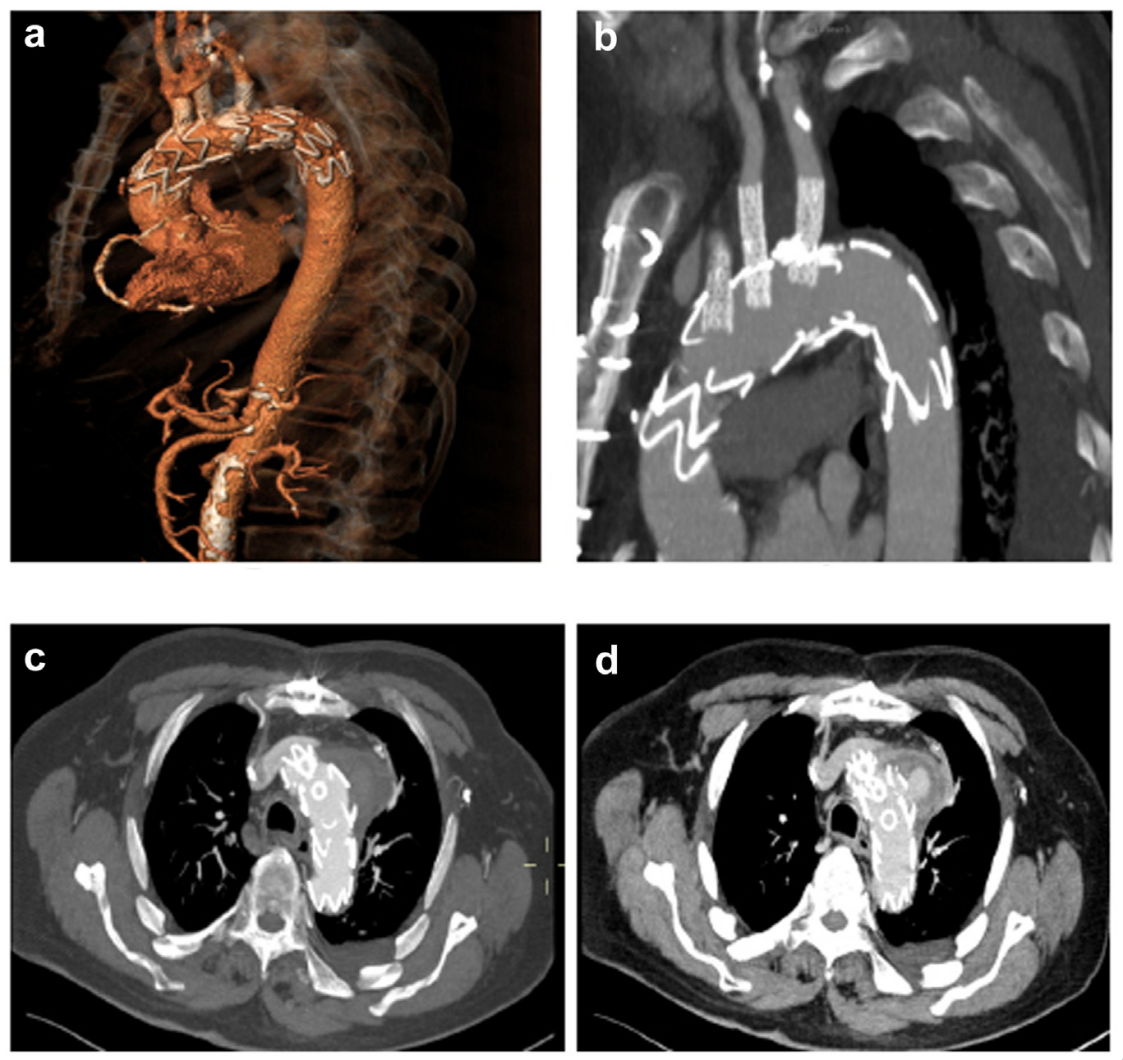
Immediate postoperative computed tomography (CT) angiography (*CTA*) scan. a, Three-dimensional reconstruction of the totally endovascularly repaired aortic arch aneurysm. b, CTA, sagittal view. c, CTA, early arterial phase axial view, with no endoleak present in the aneurysm sac. d, CTA, late venous phase axial view, showing endoleak present in the aneurysm sac.
